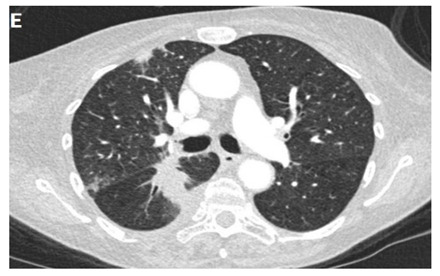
Axial CT chest (image E) showing irregular 5.5 cm mass lesion with spiculated margins in the Right Lower Lobe (RLL) with the background of emphysematous lungs denoted by an arrow.
